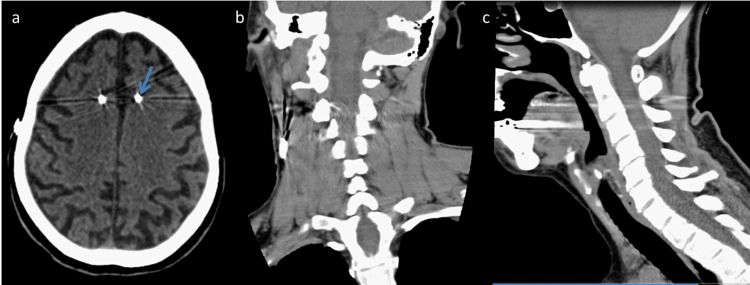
Computerized tomography (CT) of the brain showing deep brain stimulation electrodes (blue arrow) and generalized cortical atrophy (a). Cervical CT showing no structural causes of abnormal posture (b, c).
